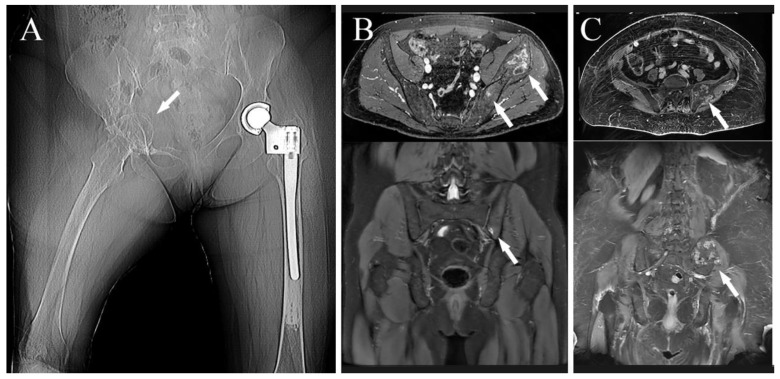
(A) Preoperative X-ray showing a right hip fixed in flection in a patient affected by metastatic hemangioendothelioma (white arrow), two years before she underwent left proximal femur replacement for pathological fracture (case 6); (B) preoperative MRI of a patient affected by Ewing’s sarcoma of the left hip involving the sacrum-iliac joint as well (white arrows) (case 7); (C) preoperative MRI showing a patient affected by a G2-chondrosarcoma of the left sacrum-iliac joint (white arrows) (case 2).
